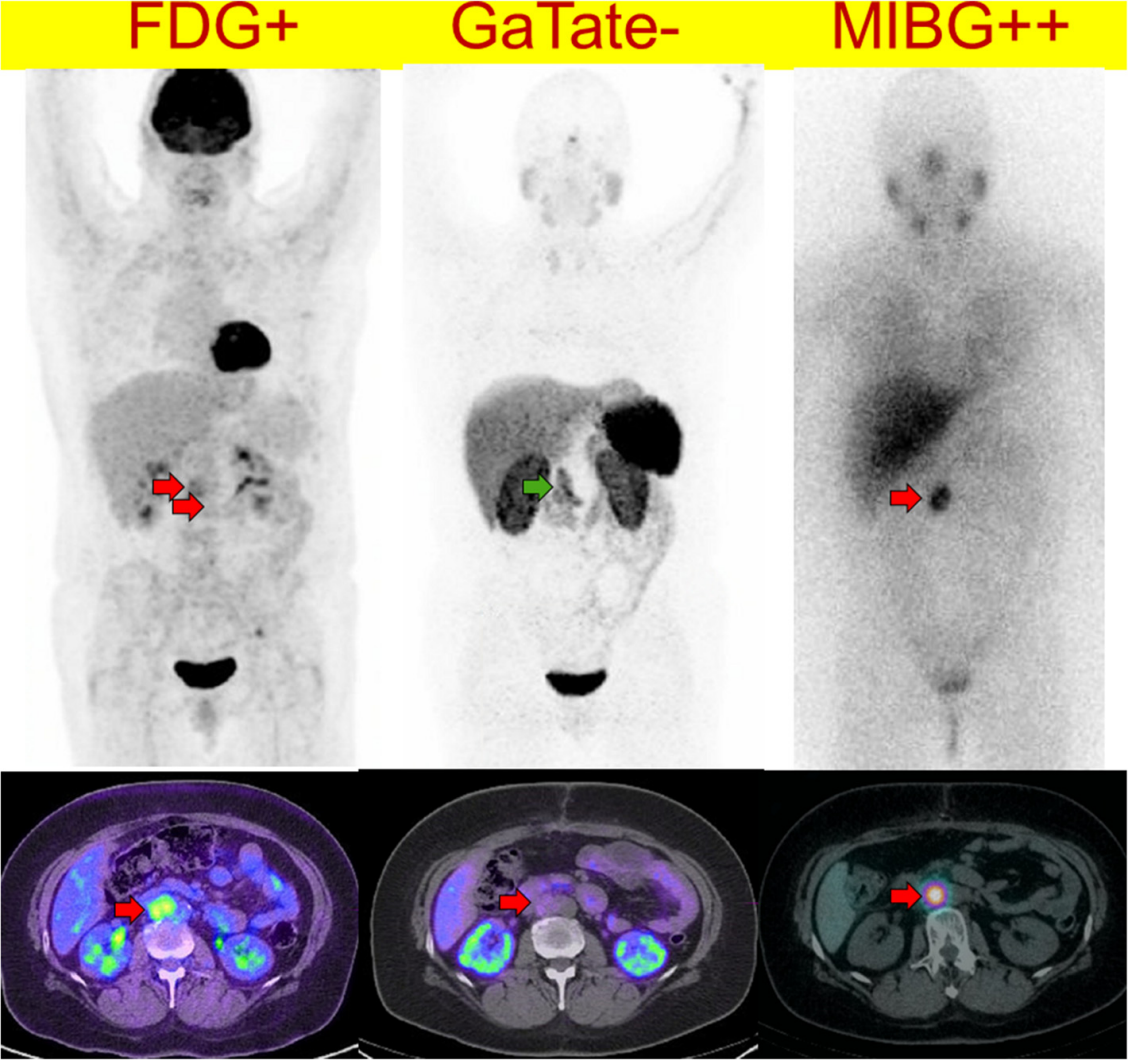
This middle-age female presented with back pain and a retroperitoneal mass on CT. F-18 FDG demonstrate moderate uptake (*left*), Ga-68 DOTATATE was negative (*middle*) with only physiologic uptake in the pancreas uncinate process (*green arrow*) while I-123 MIBG (*right*) demonstrated intense uptake. A paraganglioma was confirmed following surgical resection. Plasma and urine normetanephrine were elevated at 3728 pmol/L (*N* < 900) and 11.8 micromol/day (normal <2.3), respectively
